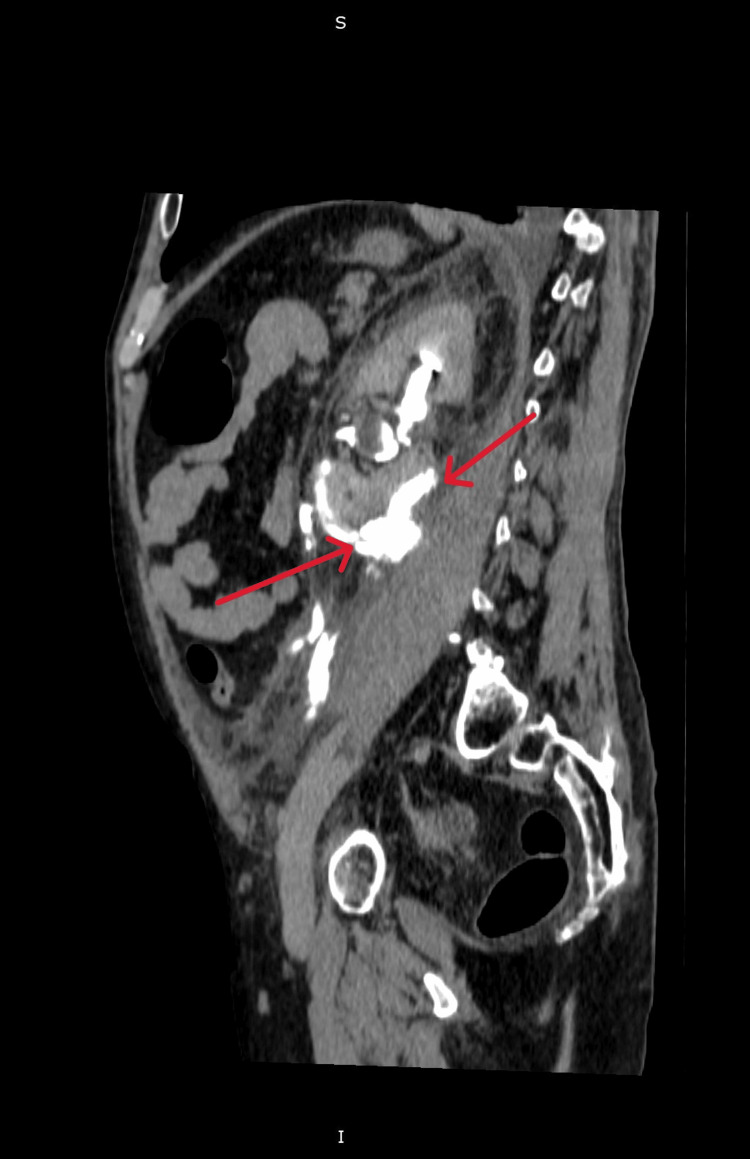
Contrast-enhanced CT image showing left hydronephrosis and large urinoma The arrows indicate the presence of urinary extravasation as well as the large perinephric collection.
